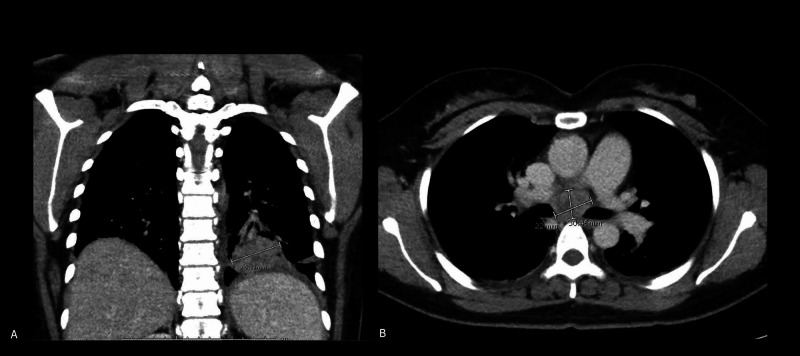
(A): CT chest coronal section showing a 4.0 X 4.1 X 3.0 cm LLL mass with (B): a subcarinal LN measuring 2.7 x 3.1 x 2.9 cm on the transverse section CT: computed tomography; LLL: left lower lung; LN: lymph node; cm: centimeter
